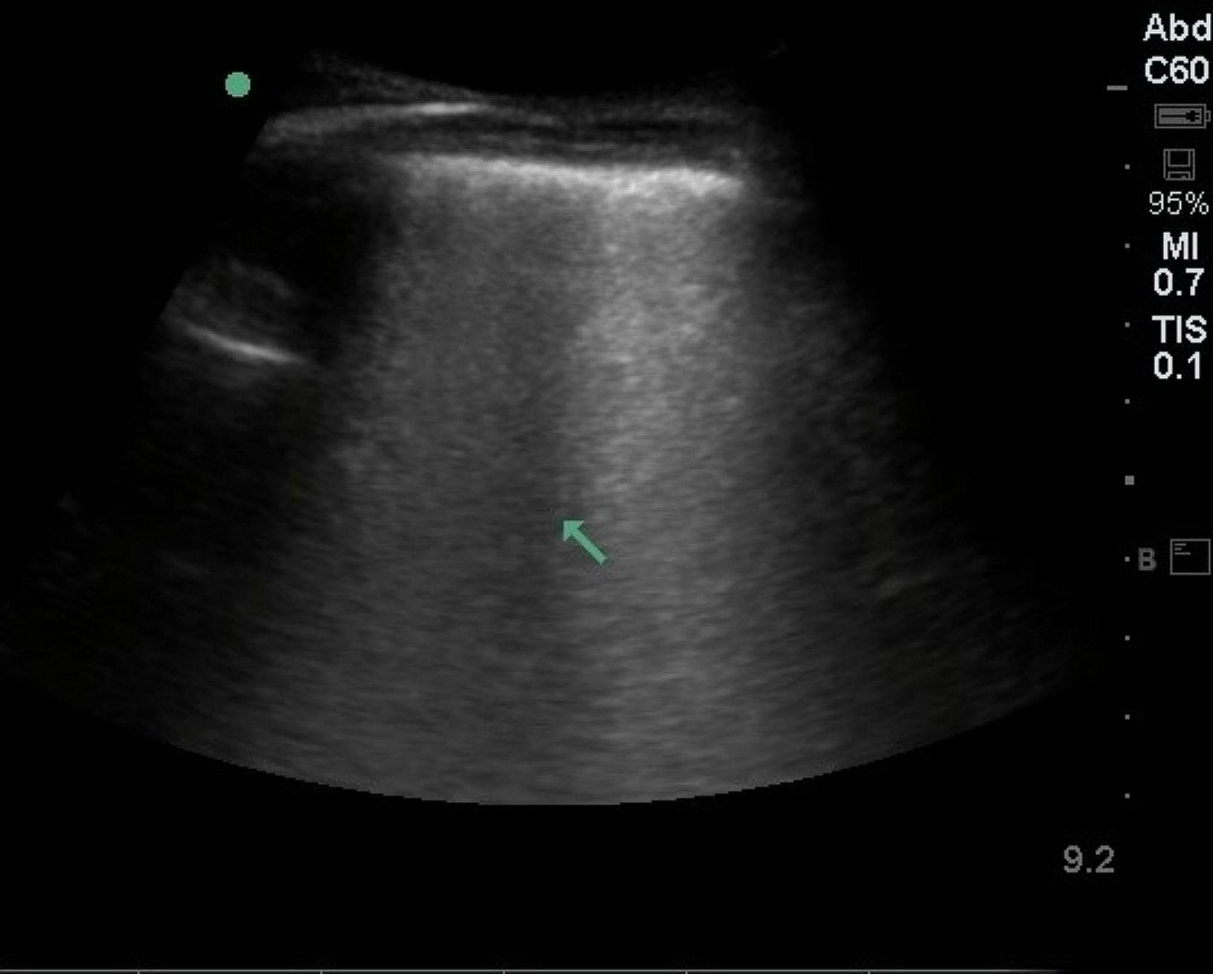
Example of a frozen loop of lung ultrasonography with extensive nine B-lines (arrow);
